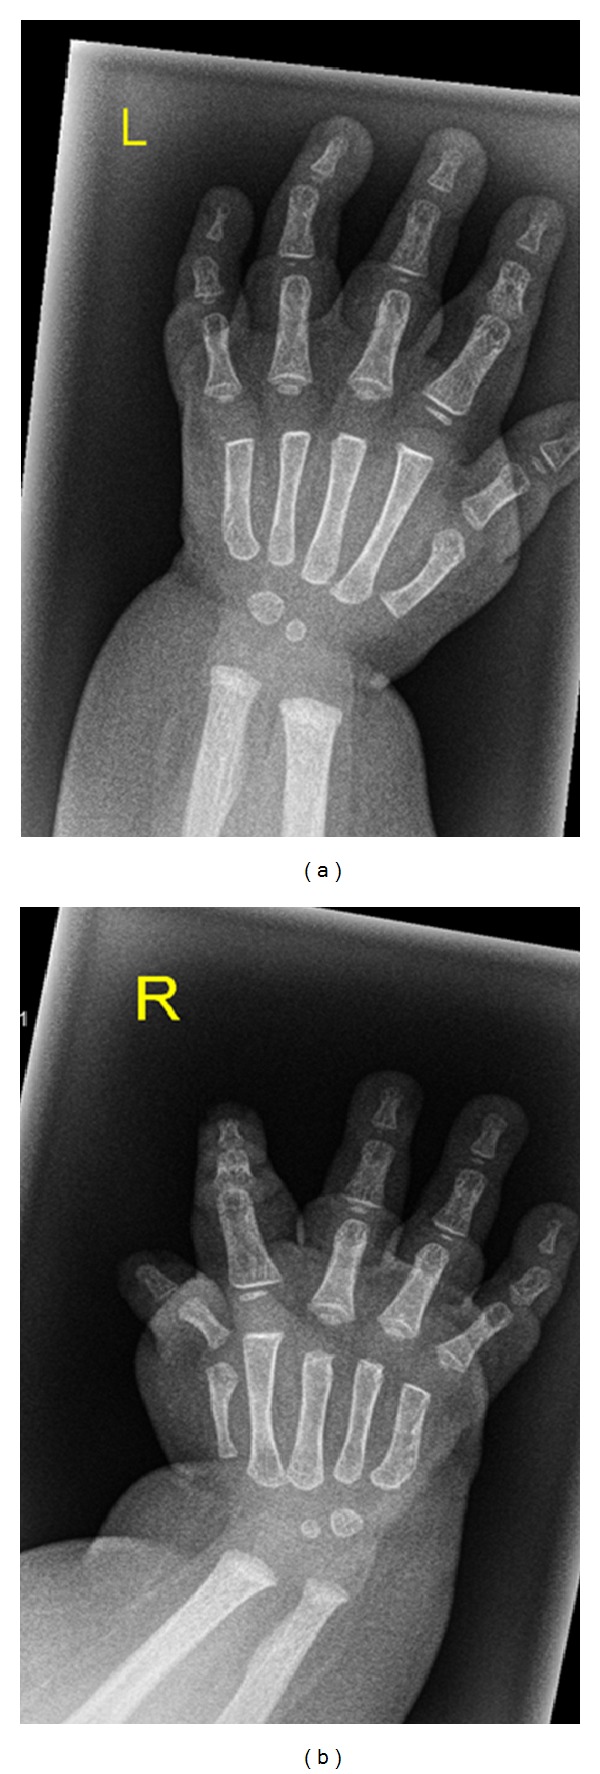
(Patient 1) Anteroposterior hand radiographs showed brachydactyly dysplastic first and second digits and clinodactyly of the 5th fingers.
